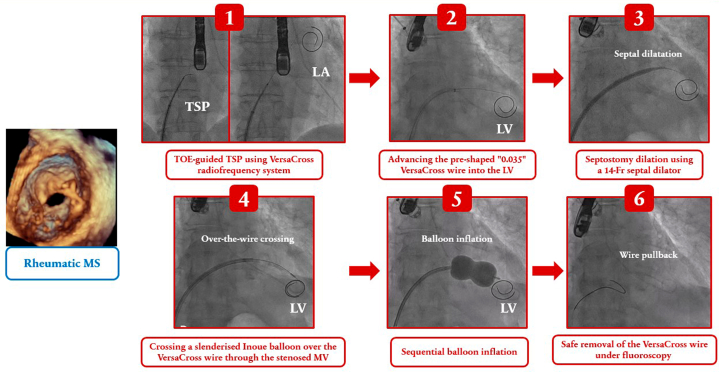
Procedural Steps of Zero-Wire Exchange PMC Using an Inoue Balloon Over the VersaCross Wire LA = left atrium; LV = left ventricle; MS = mitral stenosis; MV = mitral valve; PMC = percutaneous mitral commissurotomy; RF = radiofrequency; TOE = transesophageal echocardiography; TSP = transseptal puncture.
